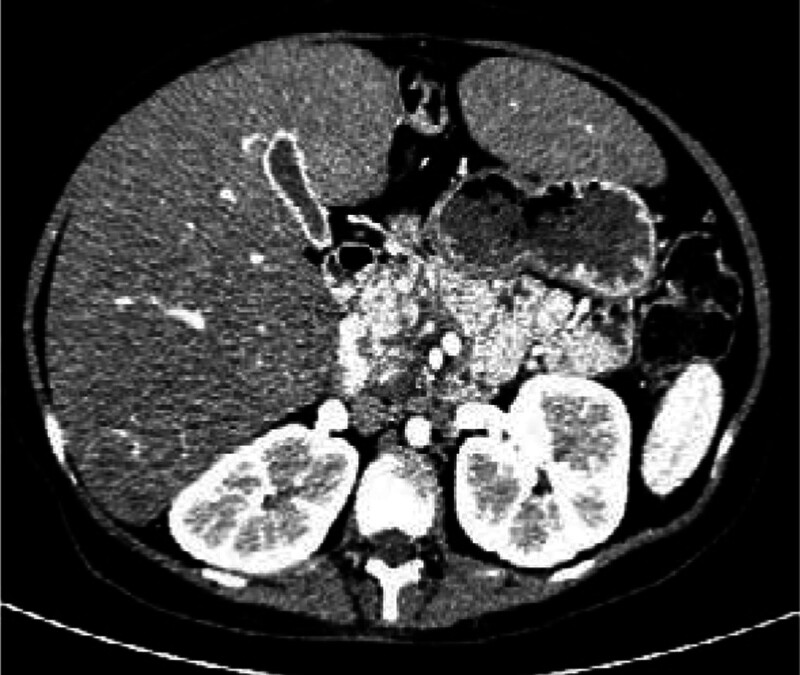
An enhanced CT scan of the upper abdomen. Unclear boundaries and increased blood vessels in the pancreas are found. CT = computed tomography.
